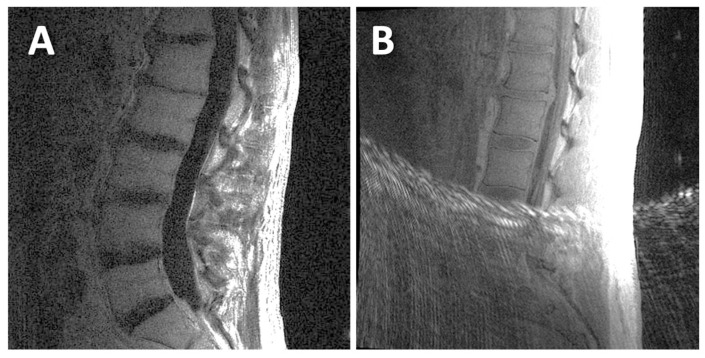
Examples of UTE MR images that were difficult to evaluate: (A) this UTE subtraction image had a low signal-to-noise ratio; (B) this UTE 1st TE image had streak artifacts that obstructed the inferior half of the image.
